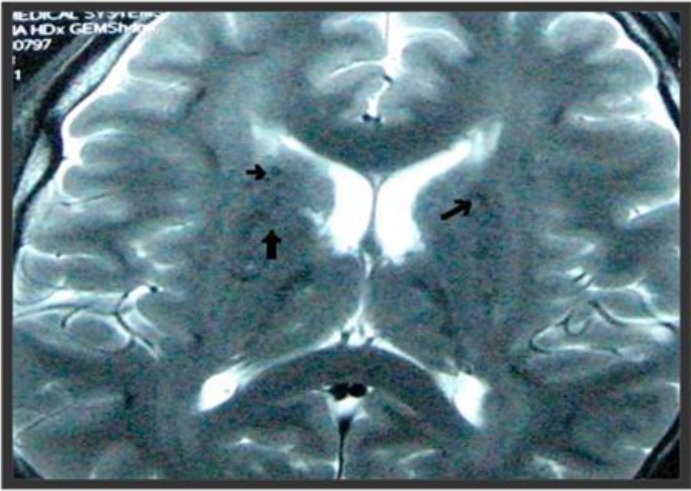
MRI of the brain showing foci of calcification (arrows) in bilateral basal ganglia and subcortical regions of bilateral cerebral hemispheres
